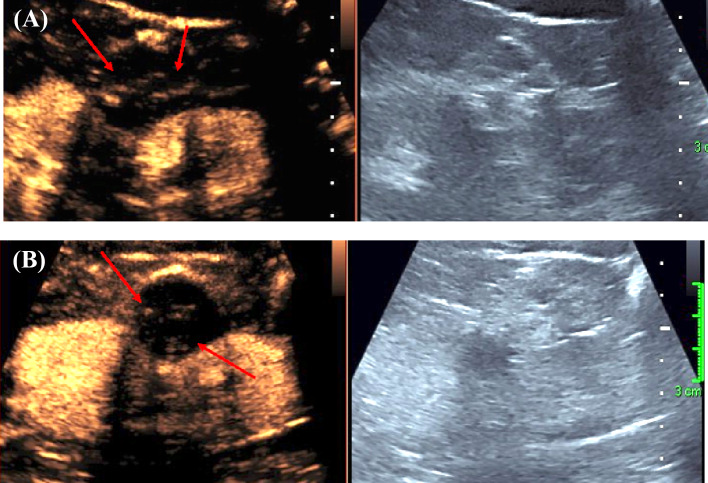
78-year-old female with enhancing septations in the ablation site post RFA of a clear cell carcinoma G1 pT1a. The CEUS is a dual screen image with the contrast enhanced ultrasound on the left and a synthesized B-mode image on the right. The B-mode image is used to confirm localization and is not a diagnostic image. On the initial post RFA CEUS (A) enhancing septations are noted (red arrows). Patient was followed and one year later (B) the enhancing septations (red arrows) are again noted and not significantly changed. The patient remains on monitoring. CECT or CEMRI were not performed
